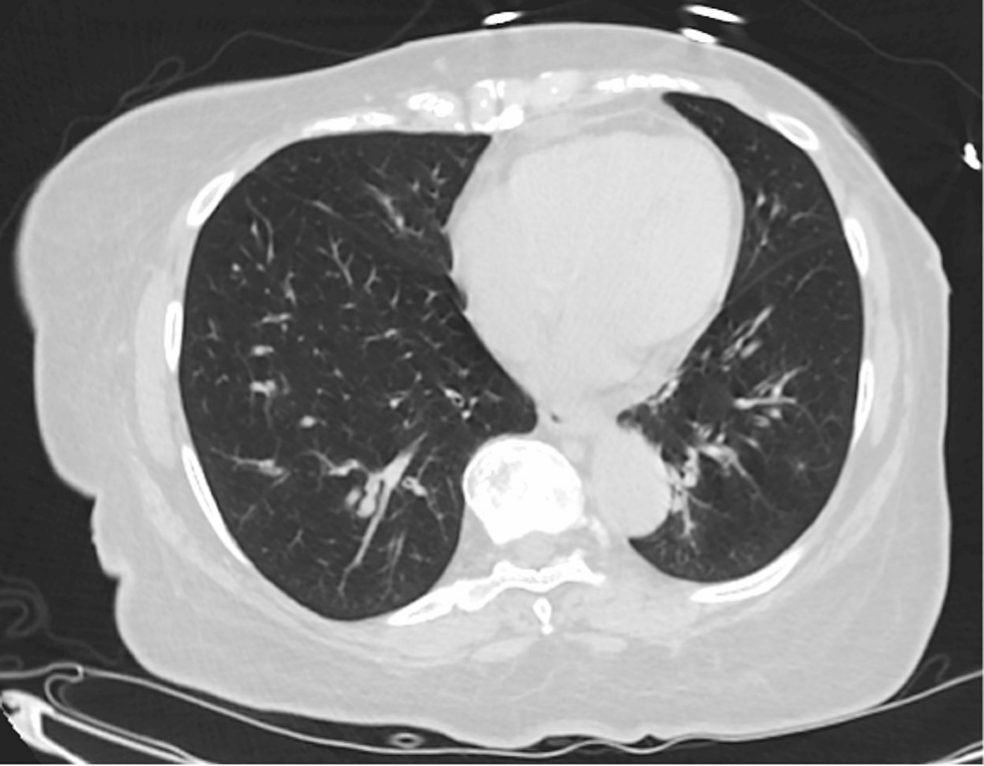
Normal chest CT. No evidence of pulmonary interstitial disease
